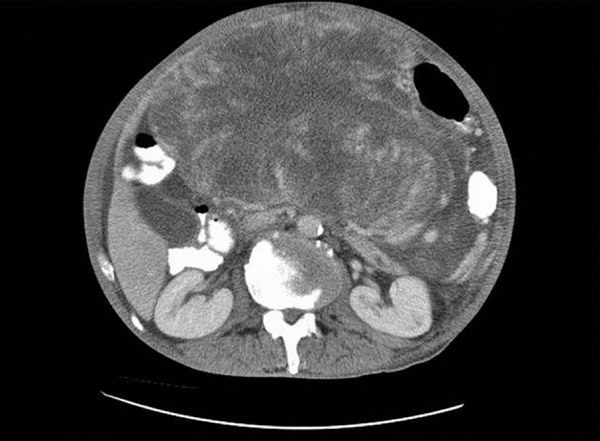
Inflammatory mass by MRI
